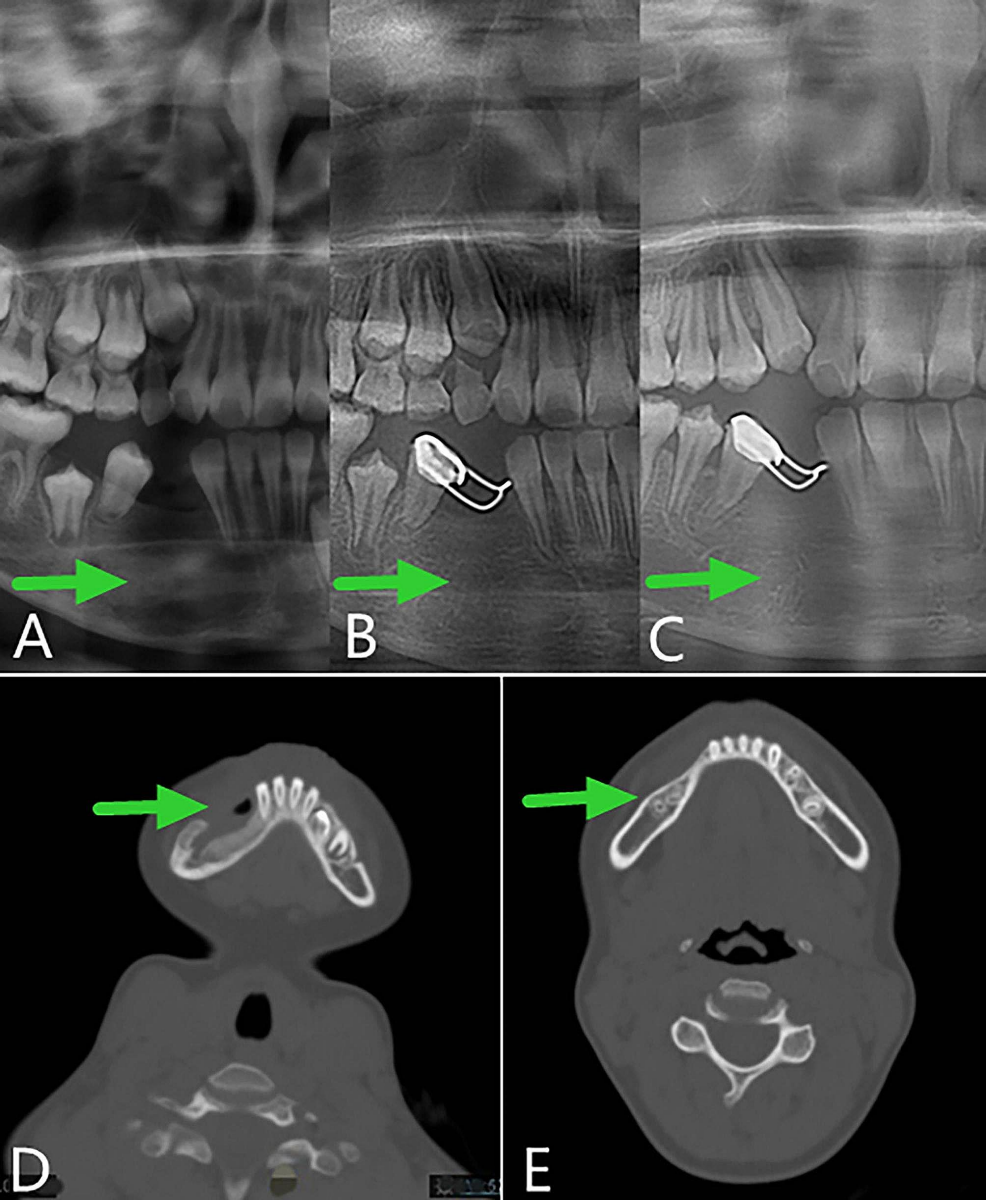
Panoramic radiography and computed tomography. A, B and C, Panoramic oral radiogram showing 2 months, 1 year and 2 years respectively after secondary curettage and fenestration closure. D and E, Computed tomography view displaying 4 months and 28 months after the curettage and fenestration
